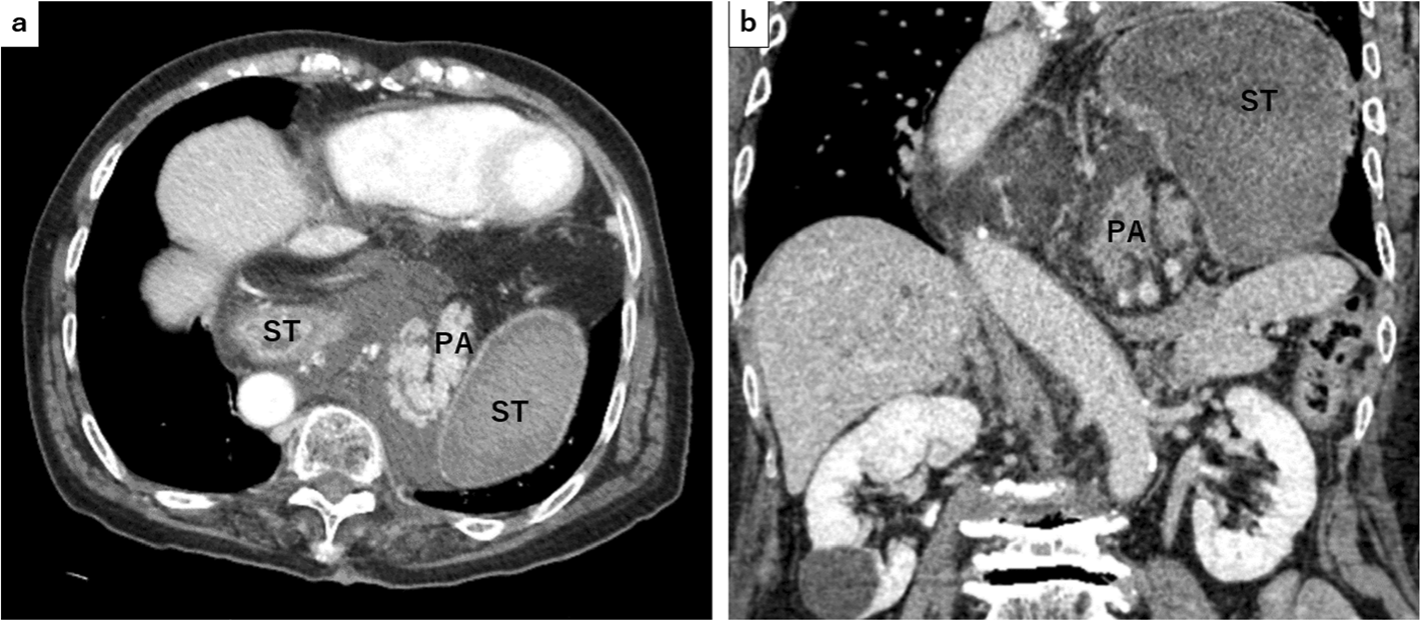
Enhanced computed tomography (eCT) revealed a large HH involving the entire stomach and pancreatic body and tail sliding through the hiatal orifice into the mediastinum, with peripancreatic fluid in the posterior mediastinal space as a sequel to pancreatitis. In axial view (a) and in coronal view (b). ST: stomach, PA: pancreas
